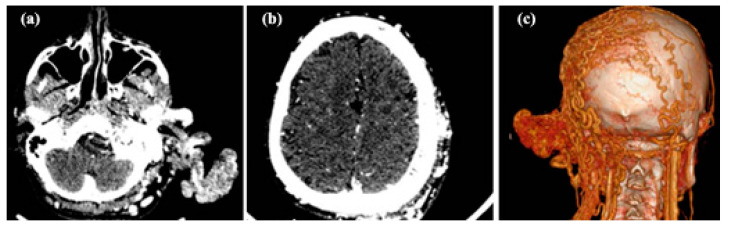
Neck CT scan (a) and cerebral CT scan (b) with contrast media and CT angiography revealing the main source of the vascular malformation from the left occipital artery and left pericrania extension (c).
